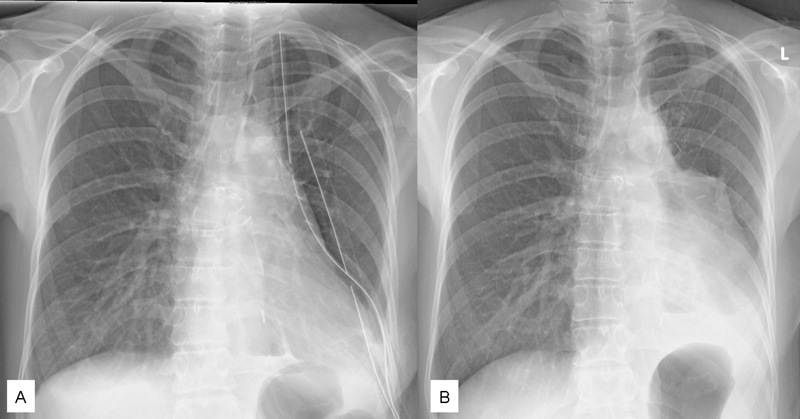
Postoperative X-ray of the chest immediately after sleeve resection of the left lower lobe (
